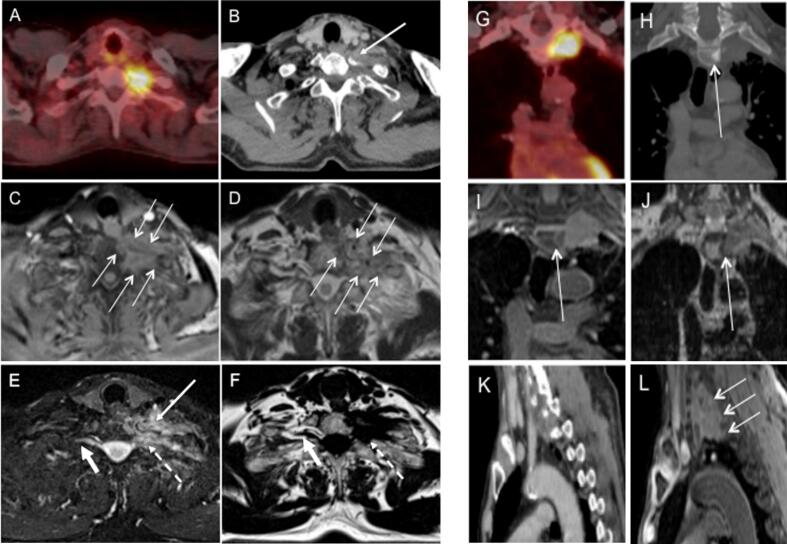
Superior sulcus tumour; (A – F). Same transverse slice of Pancoast tumour on (A) PET-CT scan, (B) CT scan (L: 46, W:600 HU) demonstrating cortical destruction (arrow), (C) fat-suppressed T_1_w Radial GRE demonstrating edges of the tumour (arrows), (D) T_2_w TSE non-fat-sat demonstrating tumour appearing darker than surrounding fat and edges of the tumour (arrows), (E) T_2_w TSE Dixon Water demonstrating tumour invasion of brachial plexus. Normal brachial plexus nerve root appears hyperintense (thick arrow). There is disruption of normal anatomy on the involved contralateral side (broken arrow) and associated hyperintense oedema (arrow) (F) T_2_w TSE Dixon Fat demonstrating tumour invasion of brachial plexus. Normal brachial plexus nerve root appears hypointense (thick arrow). There is disruption of normal anatomy on the involved contralateral side (broken arrow). (G – J): same coronal slice of a Pancoast tumour on (G) PET-CT, (H) CT scan in bone window (L:300, W:1500) showing mild hyperintensity in bone (arrow) but no obvious cortical destruction, (I) fat-suppressed T_1_w Radial GRE showing superior soft tissue extension of tumour and bone changes within the vertebral body (arrow), (J) T_2_w TSE non-fat-sat image with bone changes within the vertebral body (arrow) more pronounced than when compared to CT scan, (K and L): sagittal image on (K) CT scan showing suggestion of extension of the tumour in the intervertebral foramina and (L) fat-suppressed T_1_w Radial GRE showing more pronounced posterior extension of the tumour into the intervertebral foramina (arrows).
